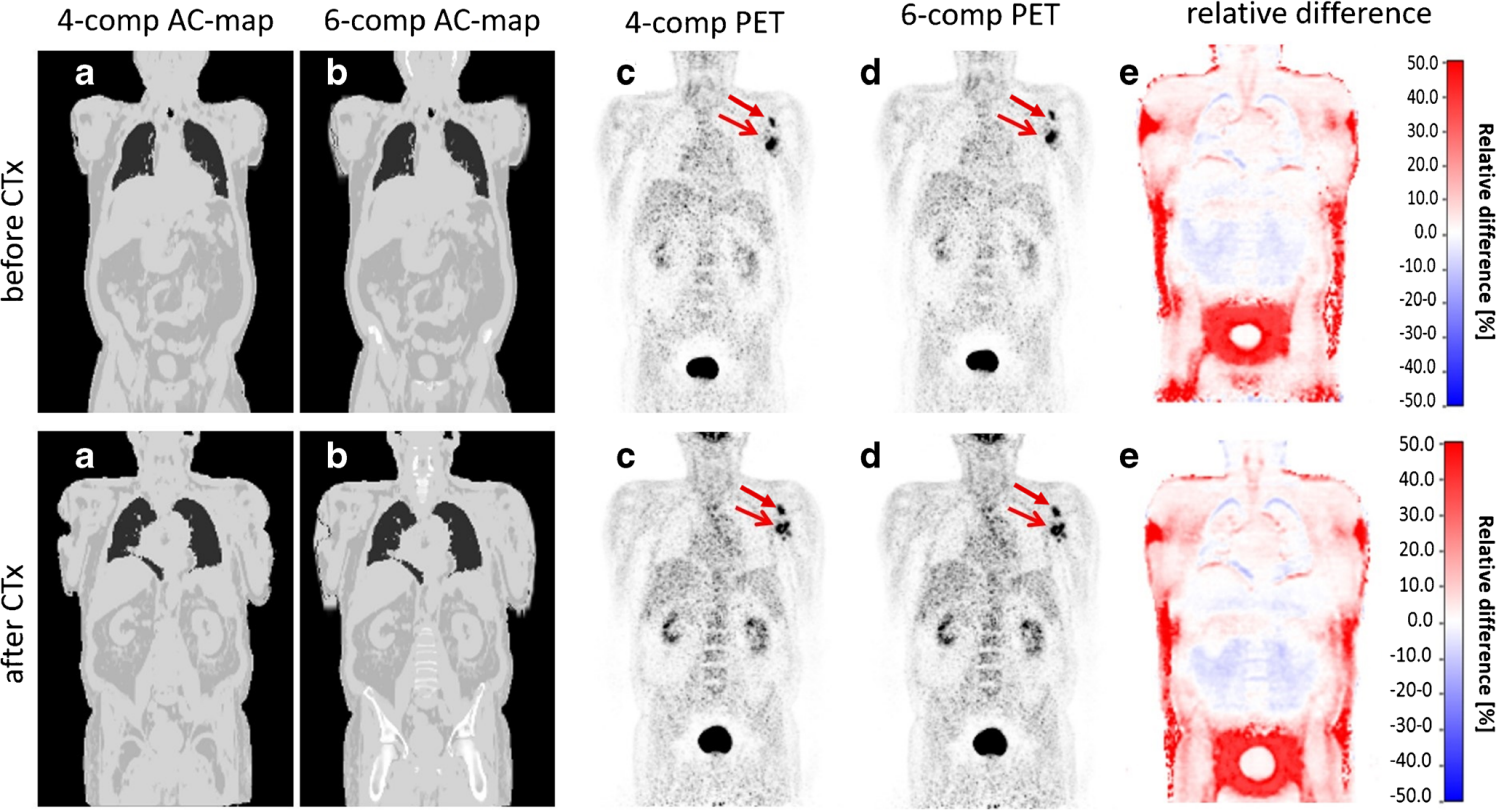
Patient case with injected ^18^F-FDG before and after chemotherapy (CTx): standard 4-compartment μ-map serving as reference (a) and improved 6-compartment μ-map (b) with corresponding corrected PET data to 4-compartments (c) and 6-compartments (d) with two marked lesions (red arrows) as well as calculated relative difference maps (e). The difference maps (e) again depict the quantitative differences between PET data reconstructed with the 4- and 6-compartment μ-maps. Note that all image panels (μ-maps, PET data, difference maps) show a high degree of comparability between both examinations before and after CTx, separated by 21 days, indicating high reproducibility. Quantitative results are provided in Table 3
